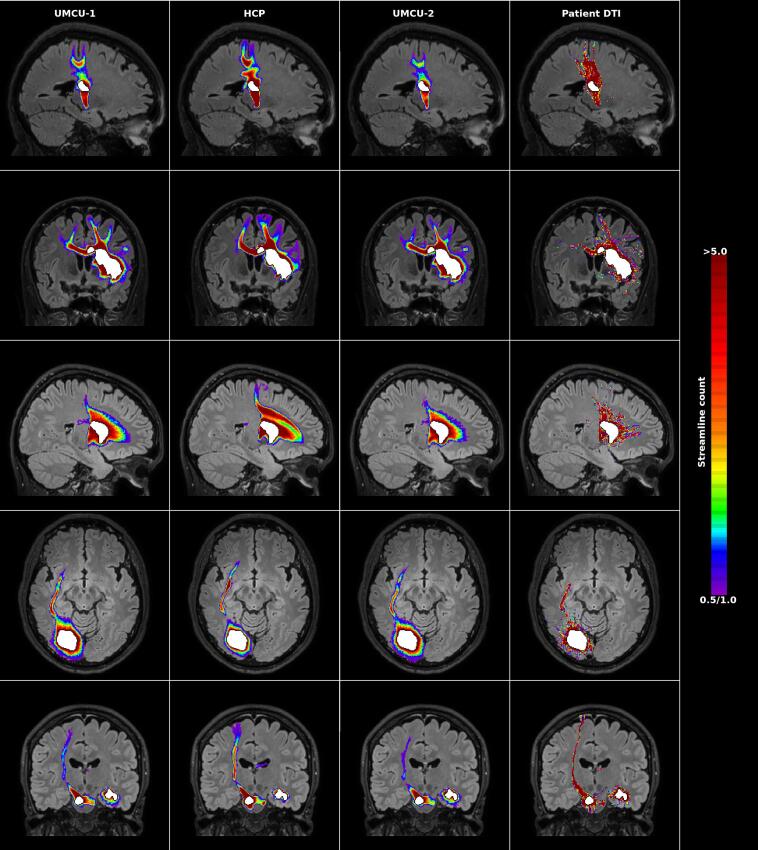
Examples of predictions of white matter damage following stroke. The left three columns depict the predictions by the three databases, where the density of damaged streamlines (count ≥ 0.5) is superimposed on the normalized FLAIR image of the individual patient. The right column shows the fiber-counts of streamlines touching the lesion, or starting in the perilesional area according to the diffusion data of the patients (count ≥ 1). Each row provides the results for a different patient. The segmented lesion is shown in white. For each patient the most informative slice is shown (i.e. the slice including on average the maximum extent of the prediction). Images are displayed according to the neurological convention.
